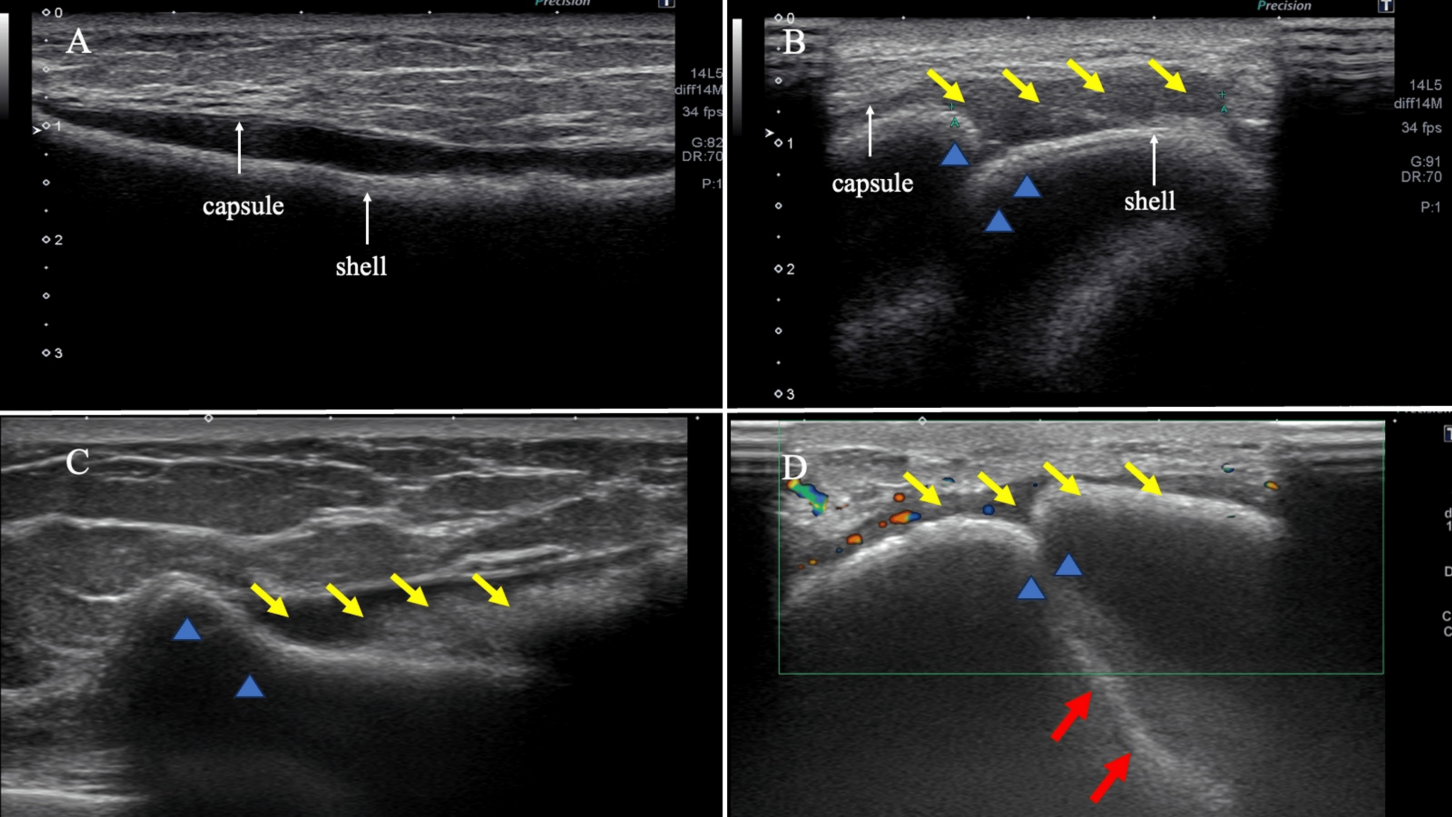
US findings three years ago (A,B) and at presentation (C,D) (A) US examination of the right breast showed separation of the outer shell and the capsule (white arrow). There was no increase in the internal echo level. (B) The left breast exhibited shell deformation (blue arrowhead) of the SBI, capsule separation from the shell, and a minor increase in subcapsular density (yellow arrow). (C) The US of the right breast at presentation showed shell deformation (blue arrow), capsule-shell separation, and a slight increase in subcapsular density (yellow arrow). (D) SBI showed hyperechoic images (red arrow) in the silicone, suggesting an extracapsular fracture in the left breast US: ultrasonography; SBI: silicone breast implant
